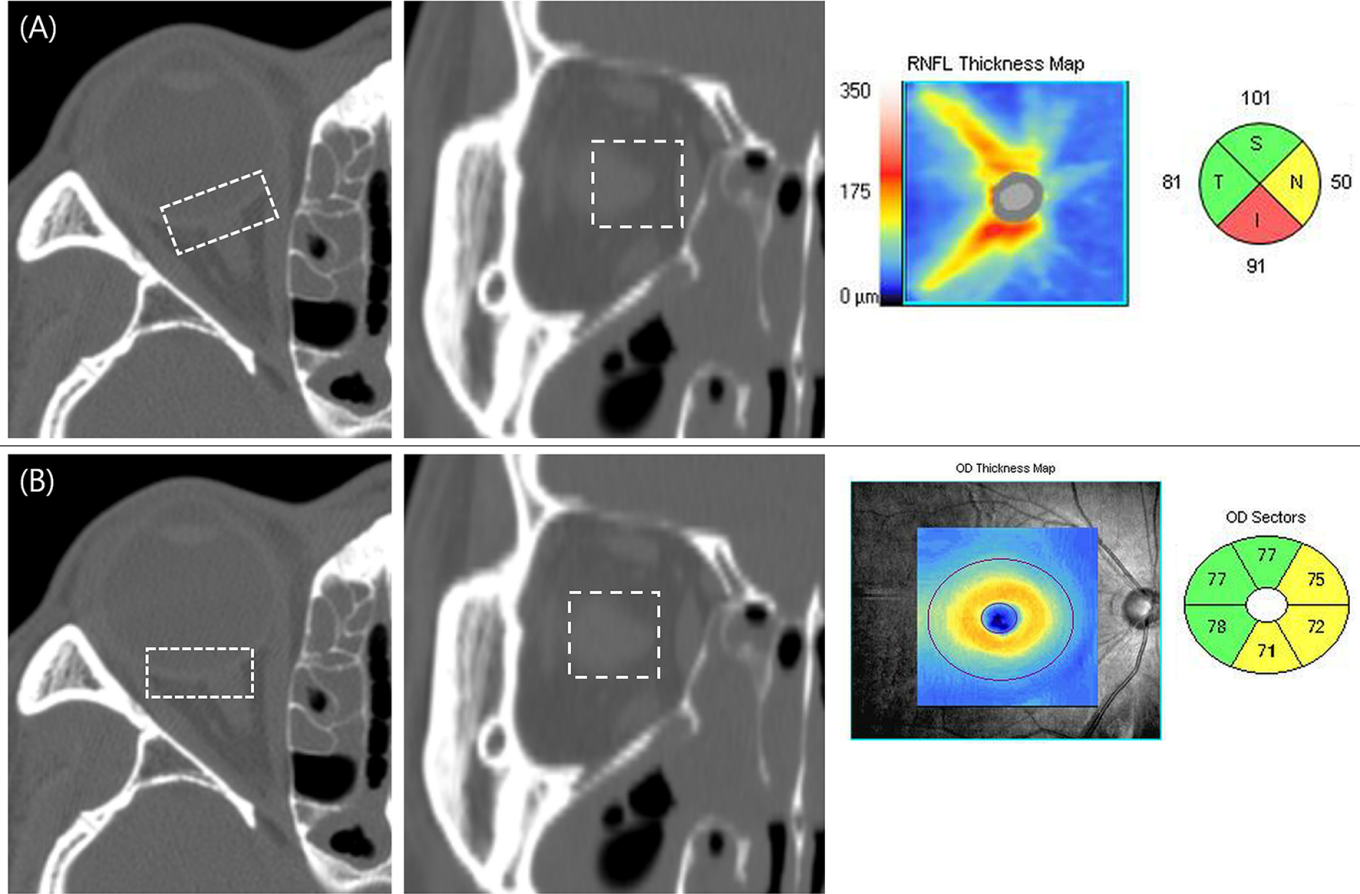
Laser interferometry scan in chronic sinusitis patients. (A) The location of the peripapillary scan with regards to the sagittal and coronal section of the ostiomeatal unit CT. OCT maps showing the retinal nerve fiber layer thickness (T = temporal, S = superior, N = nasal, and I = inferior). (B) The location of the macular scan with regards to the ostiomeatal unit CT. OCT maps showing the ganglion cell-inner plexiform layer thickness.
